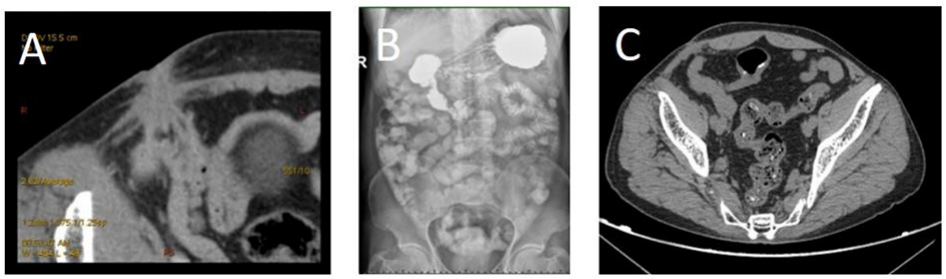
Images of representative abdominal CT and gastrointestinal radiography. (A) Infectious lesions in the right groin area, sinus formation, and involvement of the adjacent ileum; (B) no obvious signs of contrast agent leakage on barium meal imaging of the whole digestive tract; (C) 2 months after the surgery, the lesion in the right groin area is absorbed and narrowed, the sinus tract is unclear, and part of the intestinal canal in the abdominal cavity showed a metal anastomosis.
